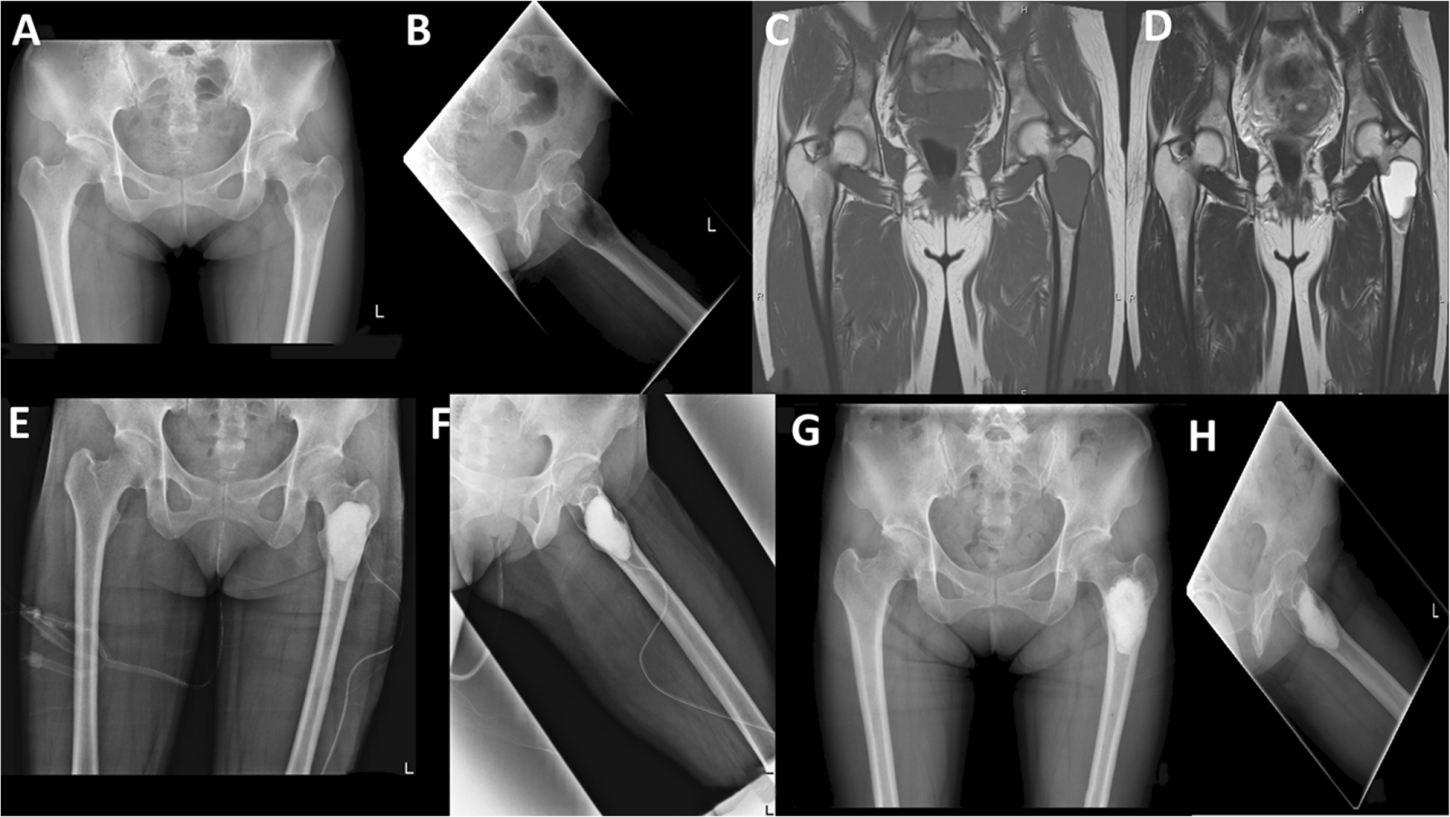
Case presentation representing a 37-year-old female with fibrous dysplasia of the left proximal femur. a, b Anteroposterior and lateral radiographs showing fibrous dysplasia of left proximal femur. c, d MRI of proximal femur showing the fibrous dysplasia lesion with marked cystic change. e, f Post-operative radiographs showing the lesion after curettage and implantation of alpha tricalcium phosphate. g, h Radiographs taken at final follow-up (4 years after surgery)
